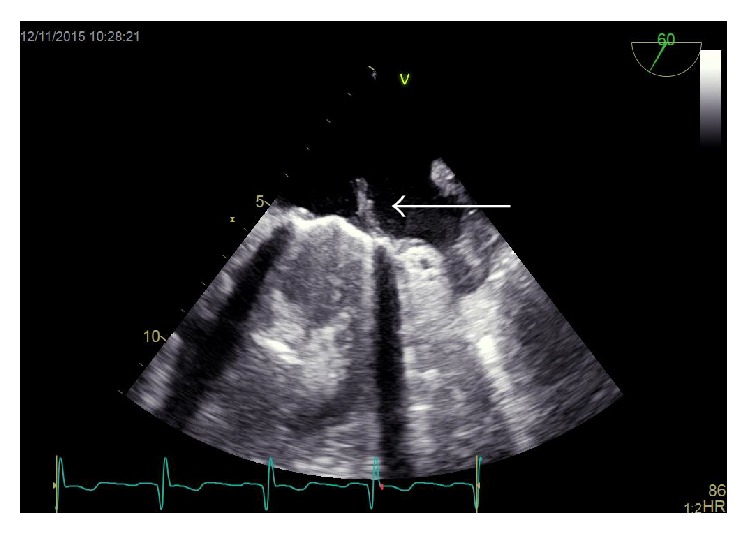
Transesophageal echocardiography of a mitral valve prosthesis. A thrombus originating from the valve ring is visible (arrow).
